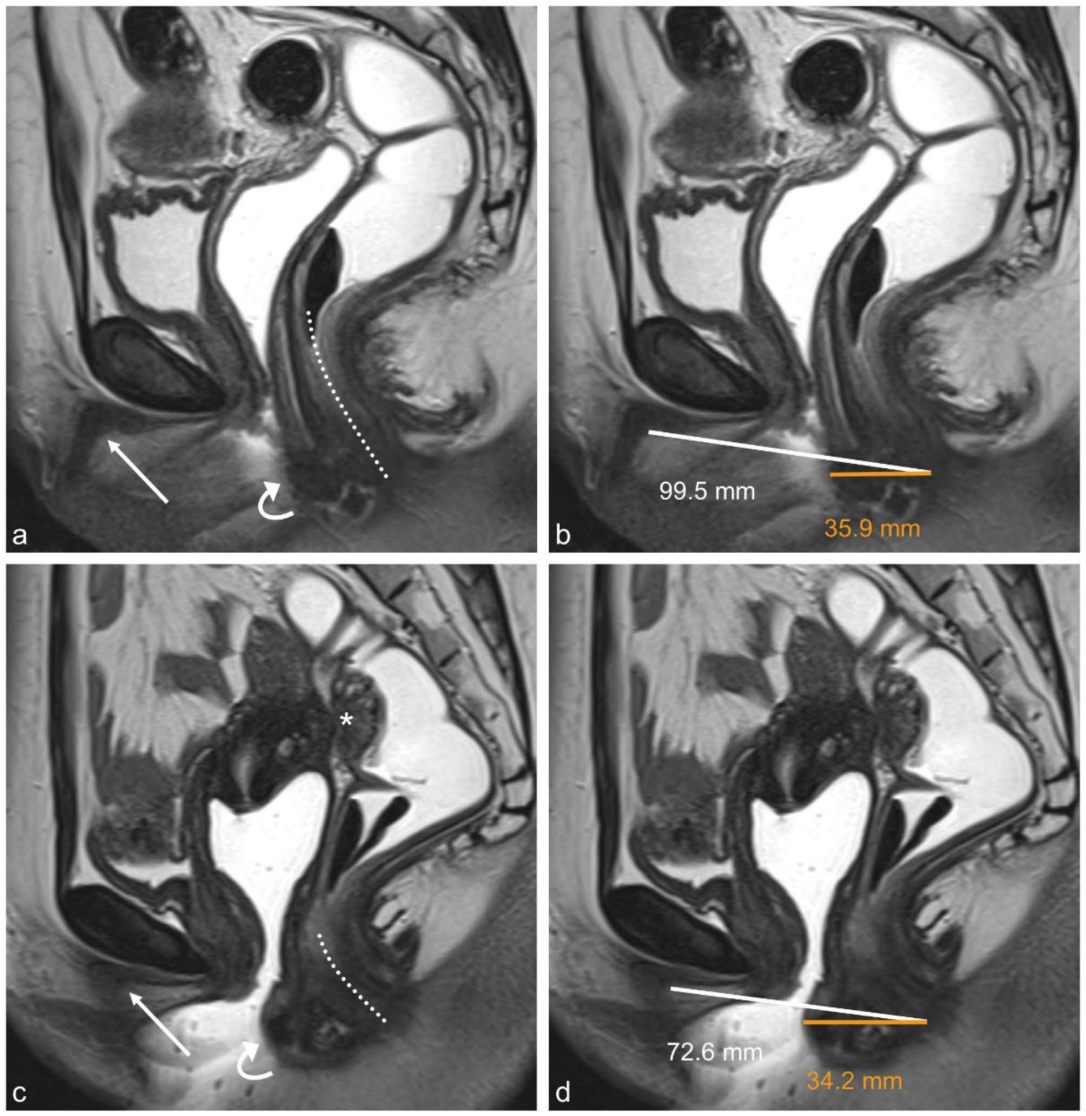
Two examples of anogenital distance (AGD) measurements in a 35-year-old woman without deep infiltrating endometriosis (DIE) (a, b) and a 31-year-old woman with DIE (c, d) on sagittal T2-weighted FSE (fast spin echo) sequences. a, c Anatomical landmarks for the measurements are the lower edge of the midline of the anal canal (dotted line), the posterior fourchette (curved arrow), and the posterior wall of the clitoris (straight arrow). b, d MRI-AGD-AC measurements from the posterior wall of the clitoris to the lower edge of the anal canal giving distances of 99.5 mm and 72.6 mm, respectively (white lines and numbers); MRI-AGD-AF measurements from the posterior fourchette to the lower edge of the anal canal giving distances of 35.9 mm and 34.2 mm, respectively (orange lines and numbers). Asterisk in c: Typical DIE with involvement of the rectum and the uterine cervix.
